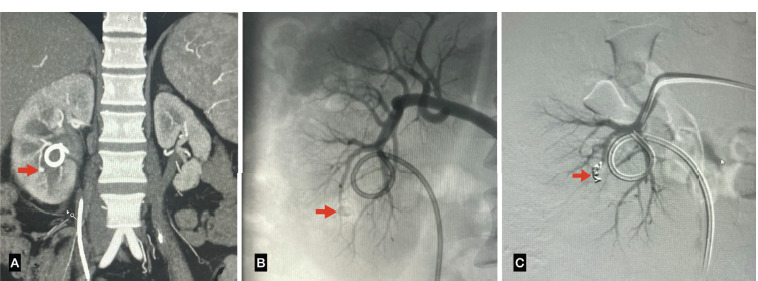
(A) CT Angiography showing a pseudo aneurysm in right lower segmental renal artery. (B) Diagnostic angiogram showing a pseudo aneurysm in the right lower renal artery. (C) Post embolisation showing a coil in the lobar artery causing causing occlusion of the pseudo aneyrysm.
